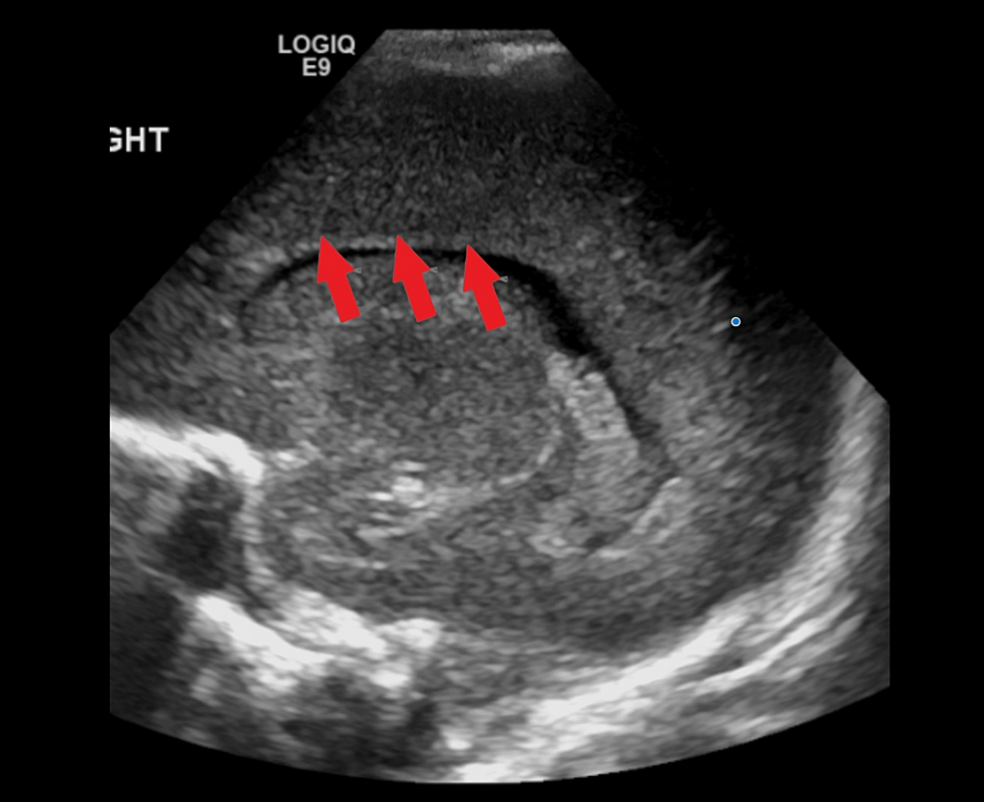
Initial transcranial ultrasound in the sagittal plane on day five showing the right hemisphere of the brain with normal findings.
